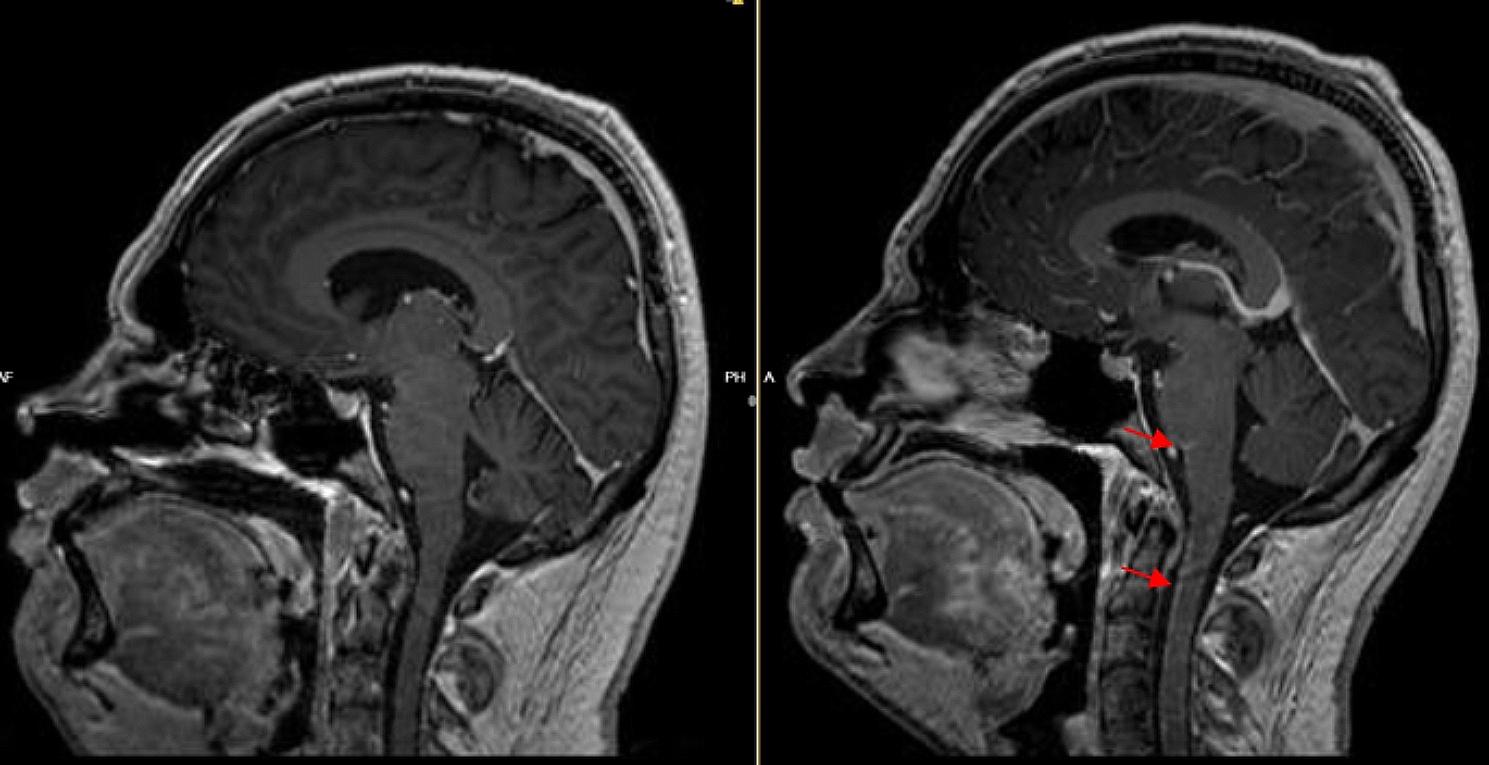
T1-weighted MRI sequences with Gadolinium enhancement. The left image demonstrates the follow-up MRI after approximately 8 weeks, with improvement of the meningeal enhancement (red arrows), pronounced at the basal brain structures and the spinal cord
